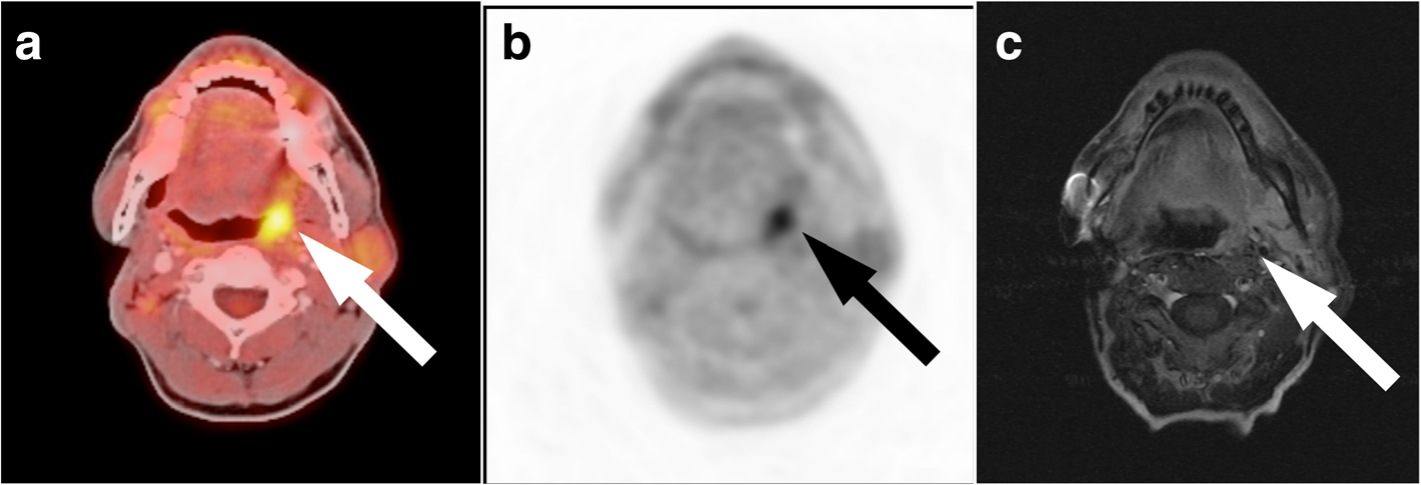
No tumour recurrence: PET/CT imaging was false-positive and MRI true-negative. Patient after resection of an ACC in the right parotid gland and mandibula. A contralateral false-positive finding is seen on the PET (b) and fused PET/CT image (a) with a focally increased FDG-uptake (SUVmax 5.4; arrow), but there was no pathological finding on the contrast-enhanced T1-weighted MR image (c)
